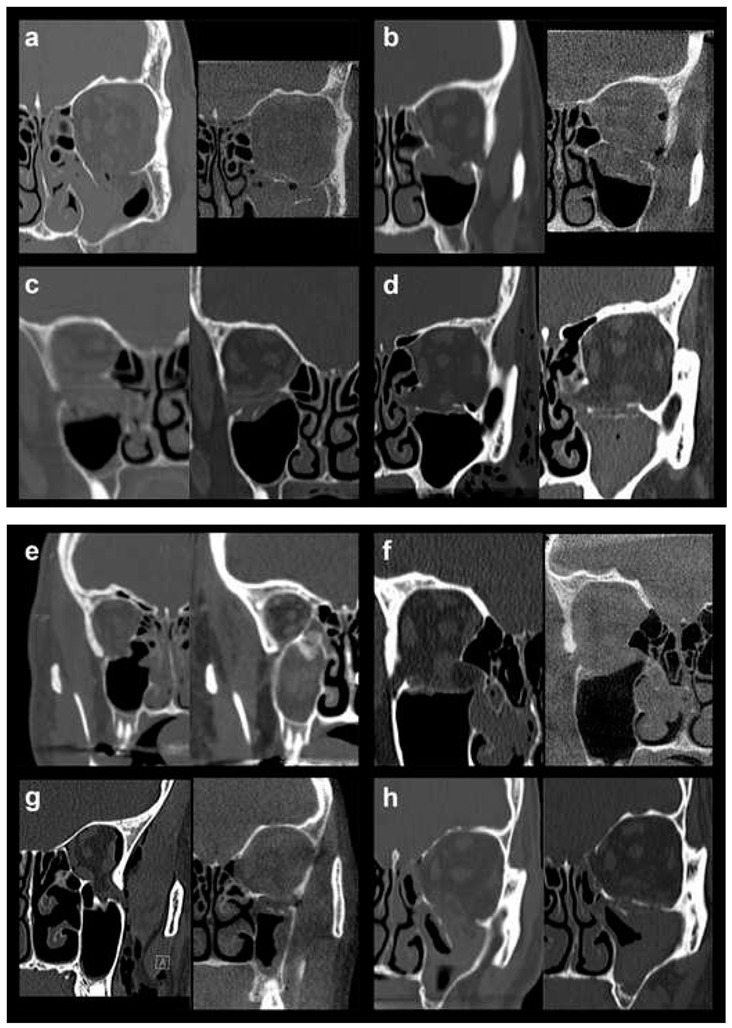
Pre- and postoperative conventional or cone-beam CT scans (coronal views) of all 7 class III fractures (a–g) and 1 class IV fracture (h).
